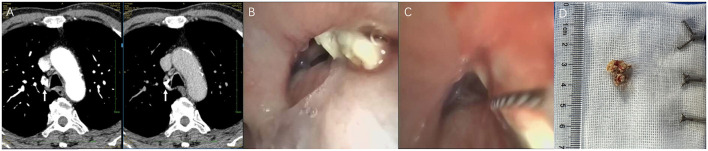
Impacted broncholith at RUL orifice. (A) CT: hyperdense focus (white arrow). (B) Bronchoscopy: broncholith wedges at RUL orifice. (C) Snare deployed after different forceps failed. (D) Retrieved 1 cm broncholith. RUL, right upper lobe; CT, computed tomography.
